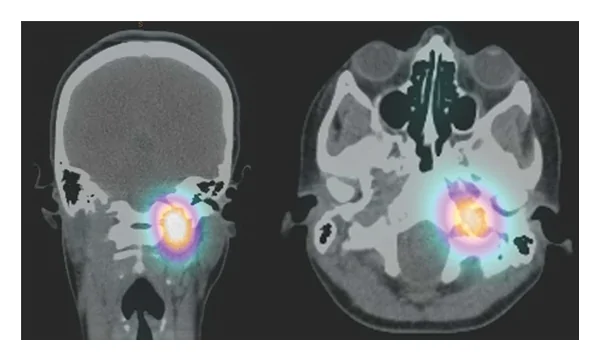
LuTate SPECT‐CT demonstrating good LuTate uptake within left glomus jugulare following the final induction cycle of PRRT.
